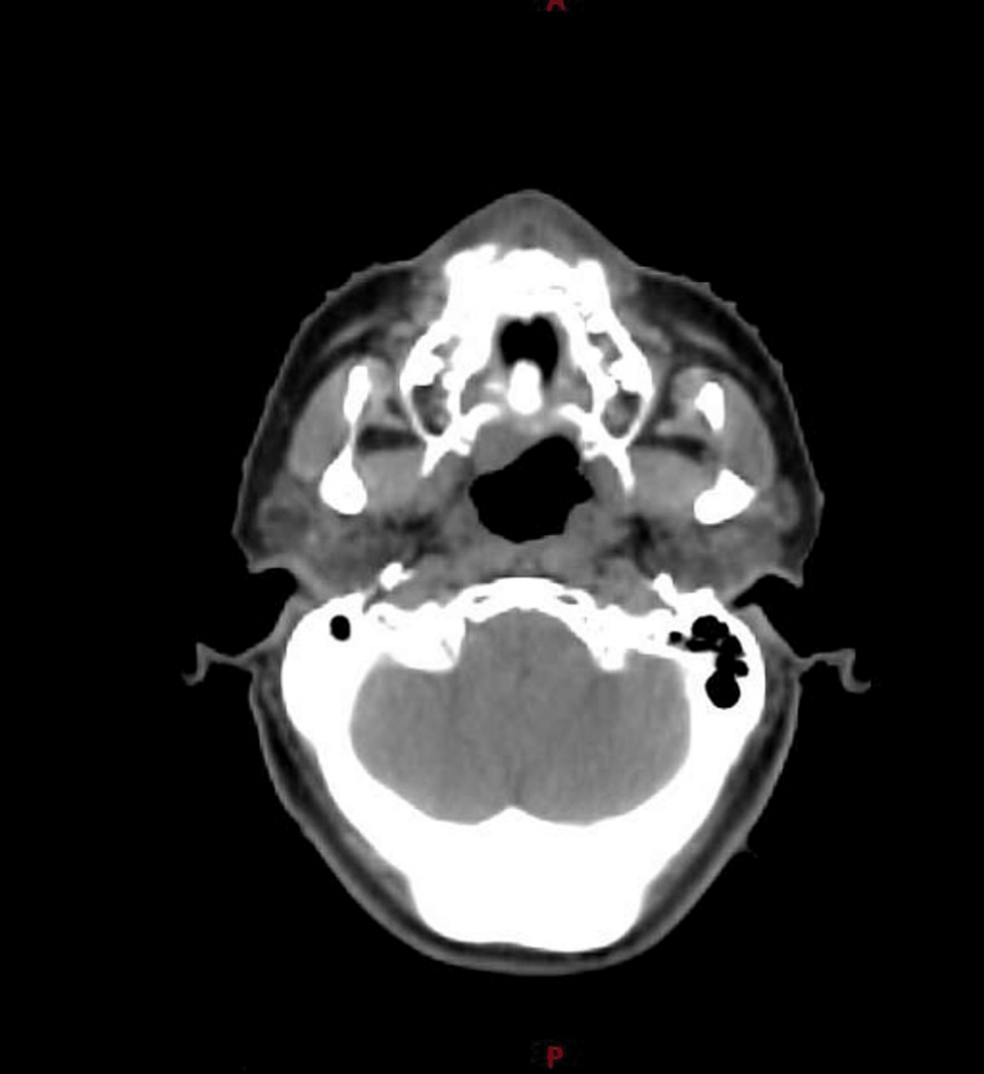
Computed tomography showing no abnormal enhancing lesions in the nasopharynx.
